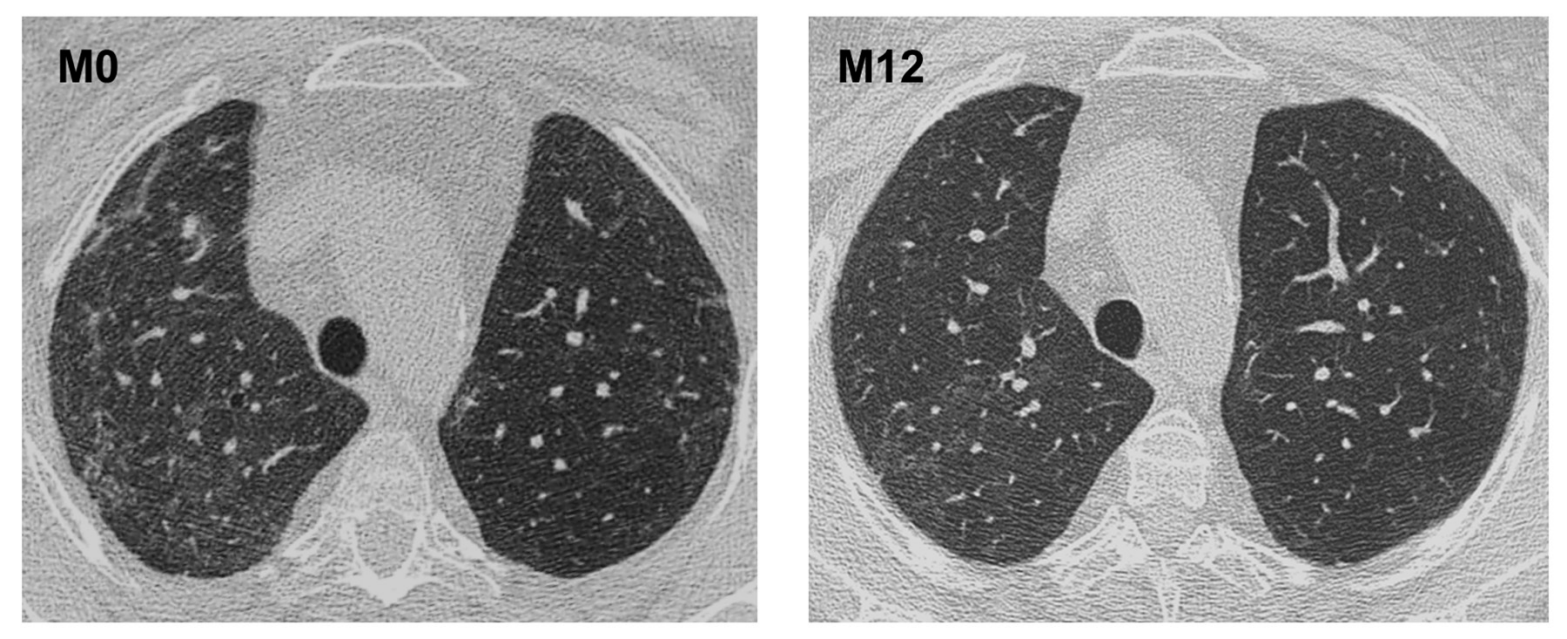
Interstitial lung disease evolution. Lung CT scans, before enrolment (M0) and 1 year later (M12), of the only patient with and nonspecific interstitial pneumonia improving after rituximab infusions.
